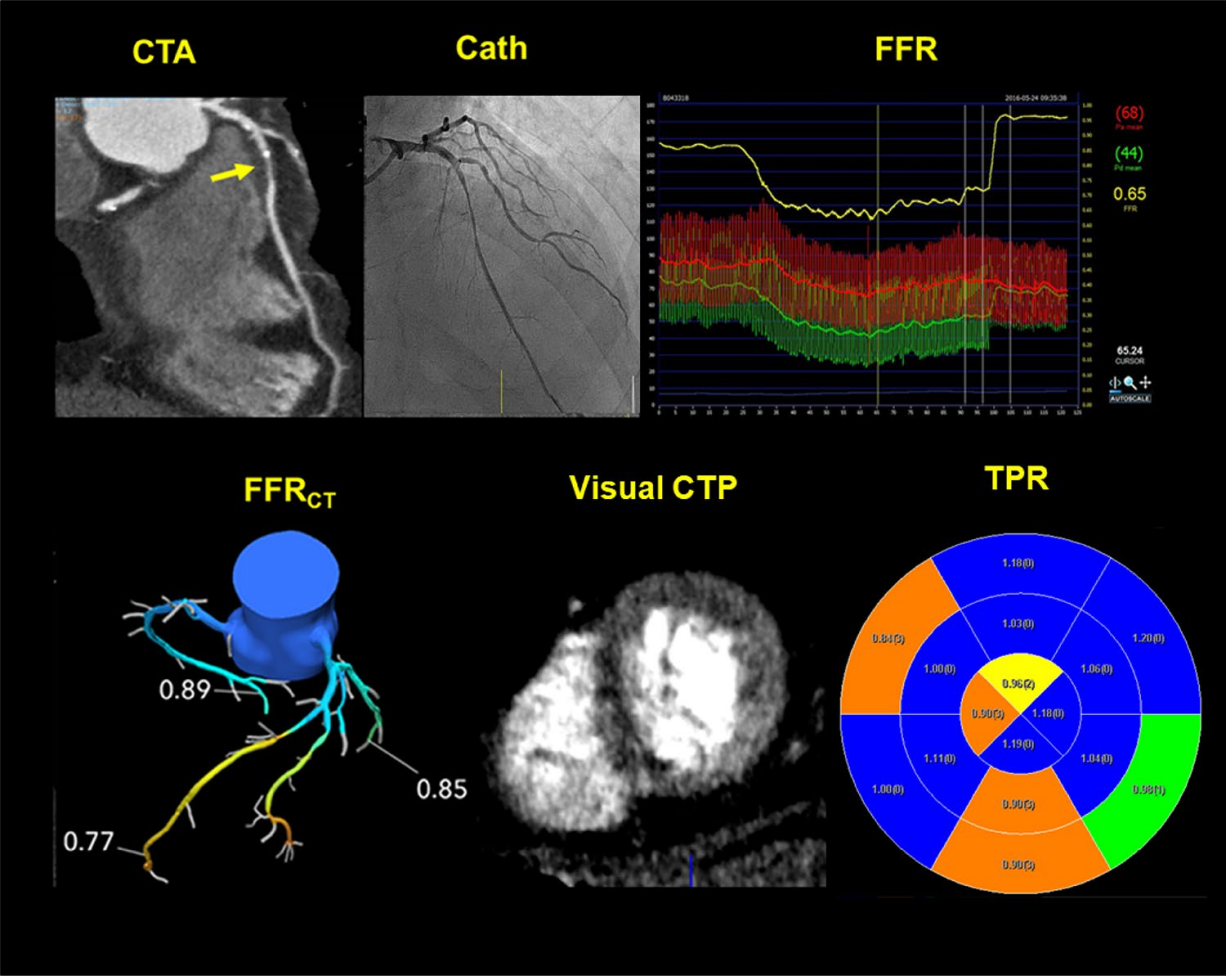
Case example. A 70 year old man with class II angina was identified to have severe mid LAD stenosis on CTA and invasive angiography with invasive FFR of 0.65. FFR was not performed in the remaining arteries which had no significant stenosis. The FFR_CT_ in LAD was 0.77. Perfusion defects were identified visually in the axial images of the myocardium corresponding to the mid anterior and septal segments. The TPR was significant in the basal and distal anterior septum (0.90)
